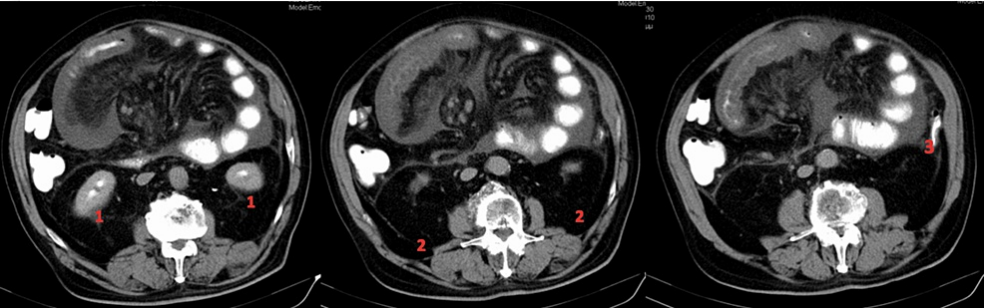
CT scan showing the lower position of the splenic flexure. The images show (1) the lower poles of the right and left kidneys, (2) perirenal adipose tissue found under the kidneys, and (3) left colic flexure (splenic flexure).
